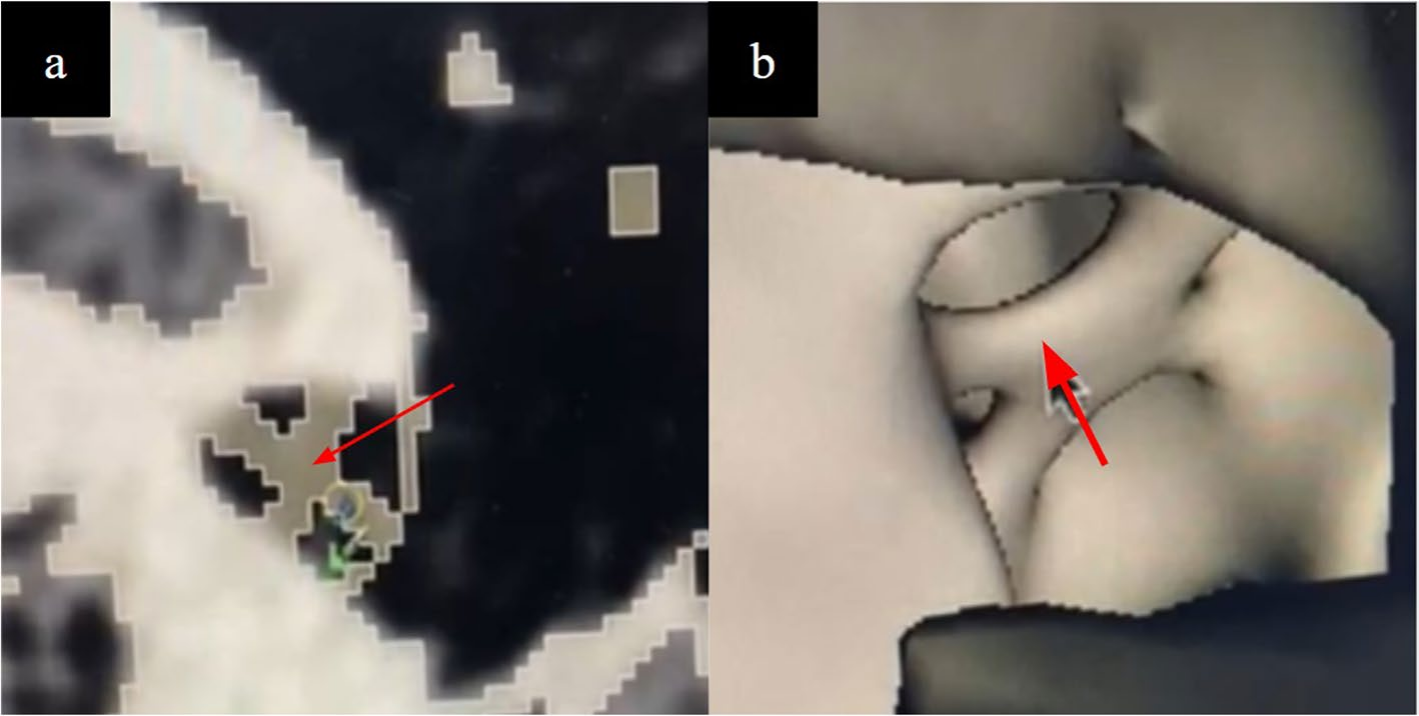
a CAD modelling and (b) 3D rendering of the round window membranous opening depicted with an X-shaped structure, which although non-anatomical serves to delineate its location perpendicular to the opening of the oval window and both crus of the stapes. This is not well visualised on CT due to its thin diameter and low density
